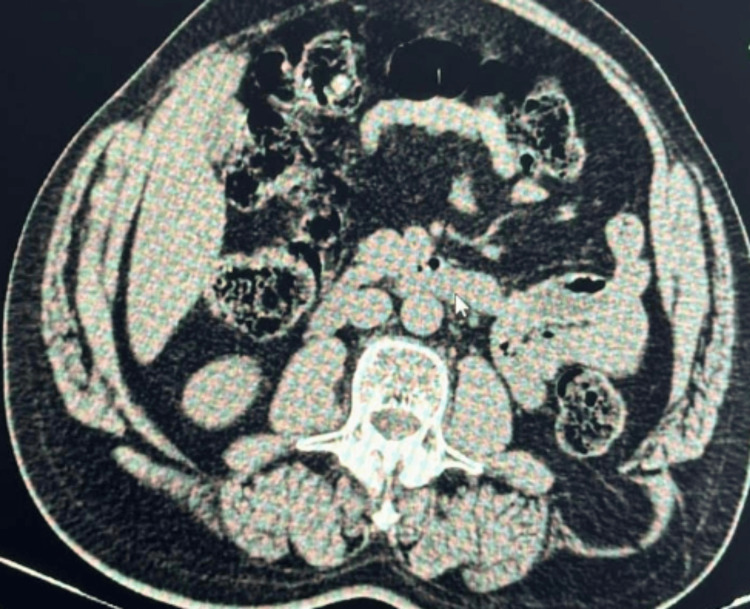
CT scan of the abdomen (axial section) reveals a defect in the left lumbar region within the superior lumbar triangle. This defect is associated with the herniation of perinephric fat through the posterior abdominal wall, confirming a lumbar hernia. A defect measuring approximately 2 cm in the left lumbar region with herniation of perinephric fat through the defect.
